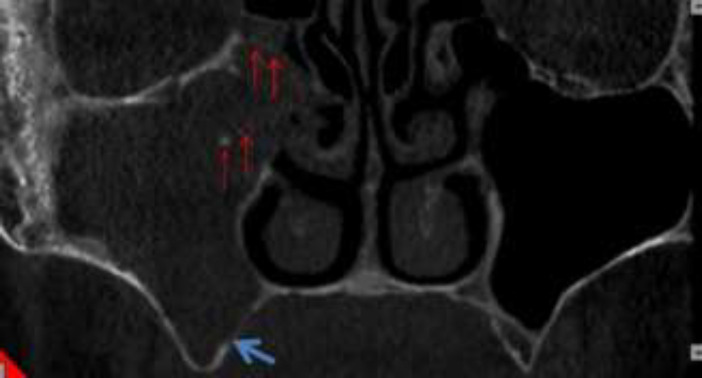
Coronal view of CBCT showing complete opacification of right maxillary sinus with calcified particles (red arrows). Notice Invasion to lateral wall of middle turbinate and the right OMC was blocked. Also, there was noticeable of palatal bone erosion (blue arrow)
